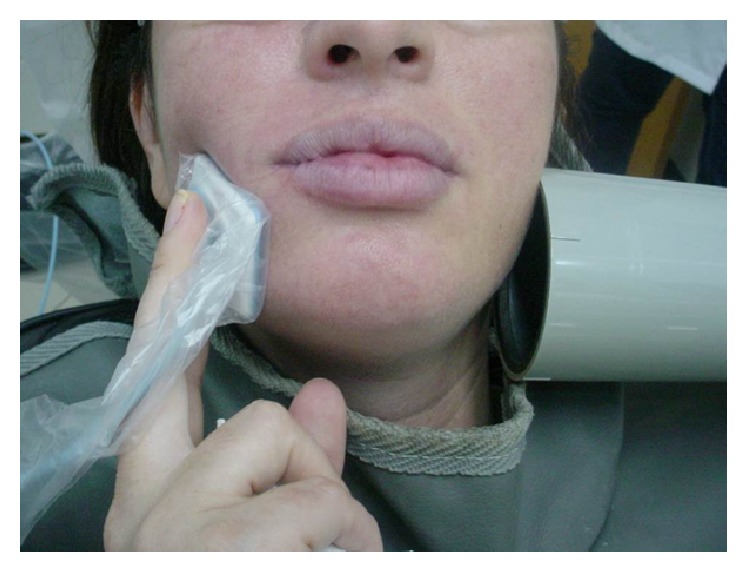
Extraoral technique used for final radiograph after root canal therapy.
